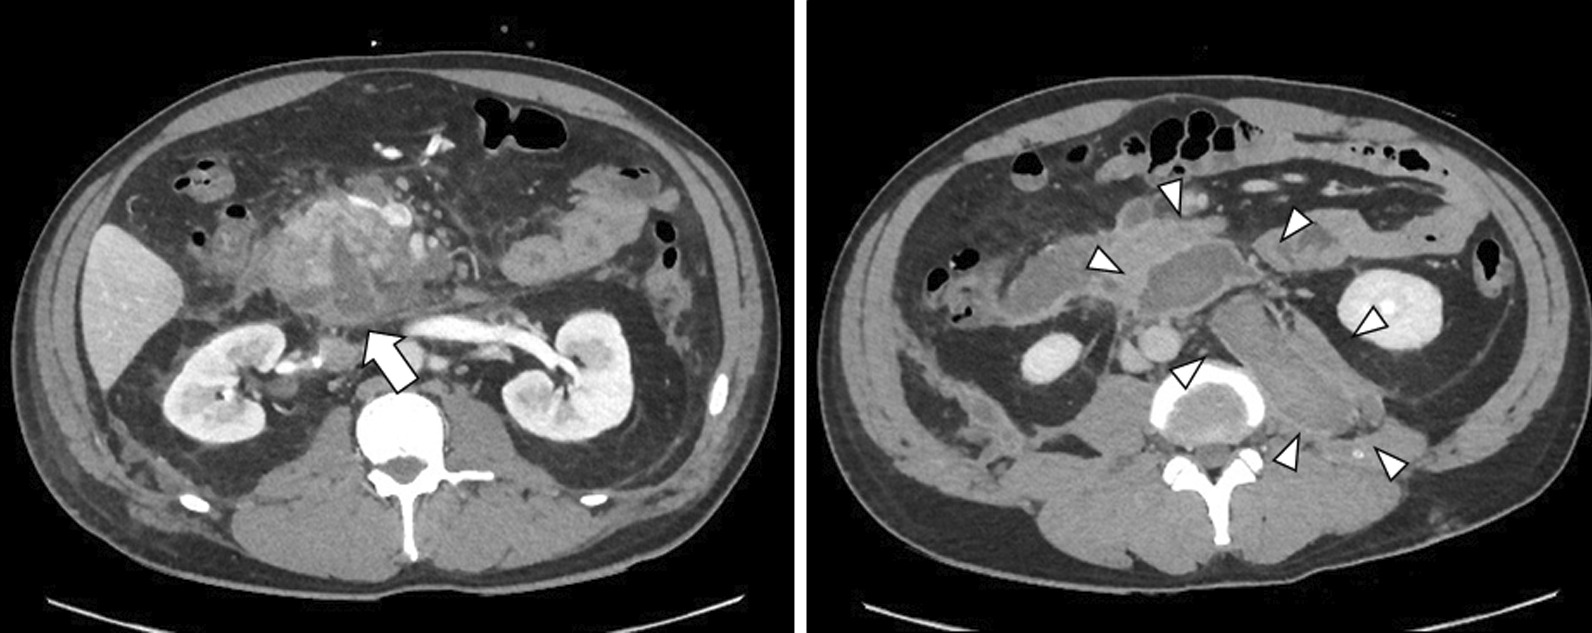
Computed tomographic image showing a wedge-shaped, low-density area in the pancreatic head (arrow), and consecutive pseudocysts behind the pancreas and in the left psoas muscle (arrowheads).
